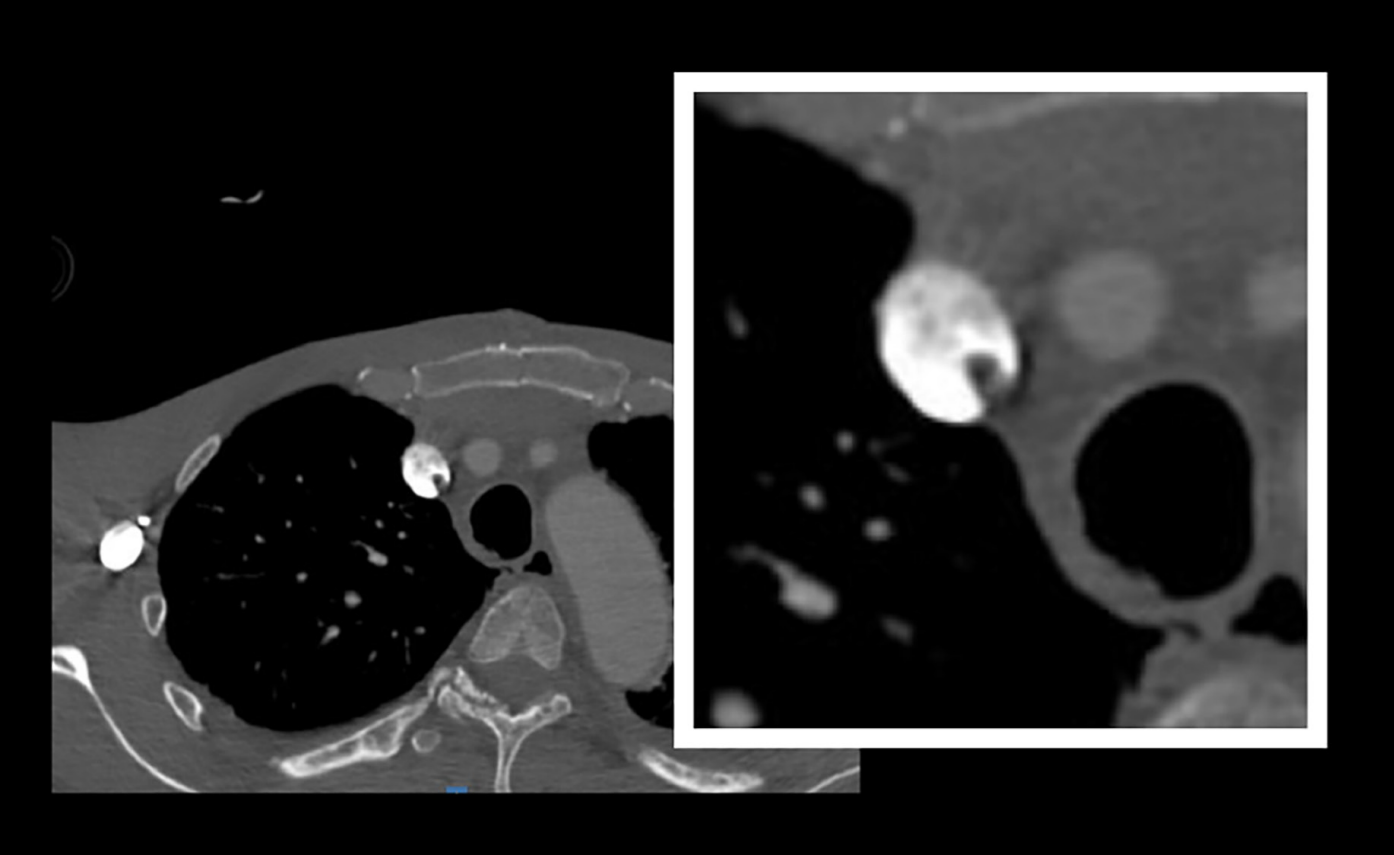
CTA demonstrating fibrin sheath with intraluminal and extraluminal air.
